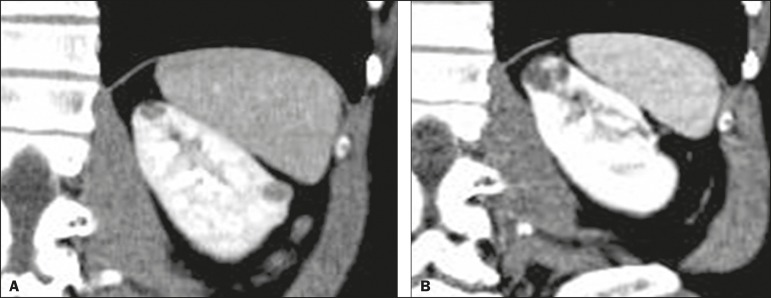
Abdominal CT scan, in a coronal view after intravenous contrast
